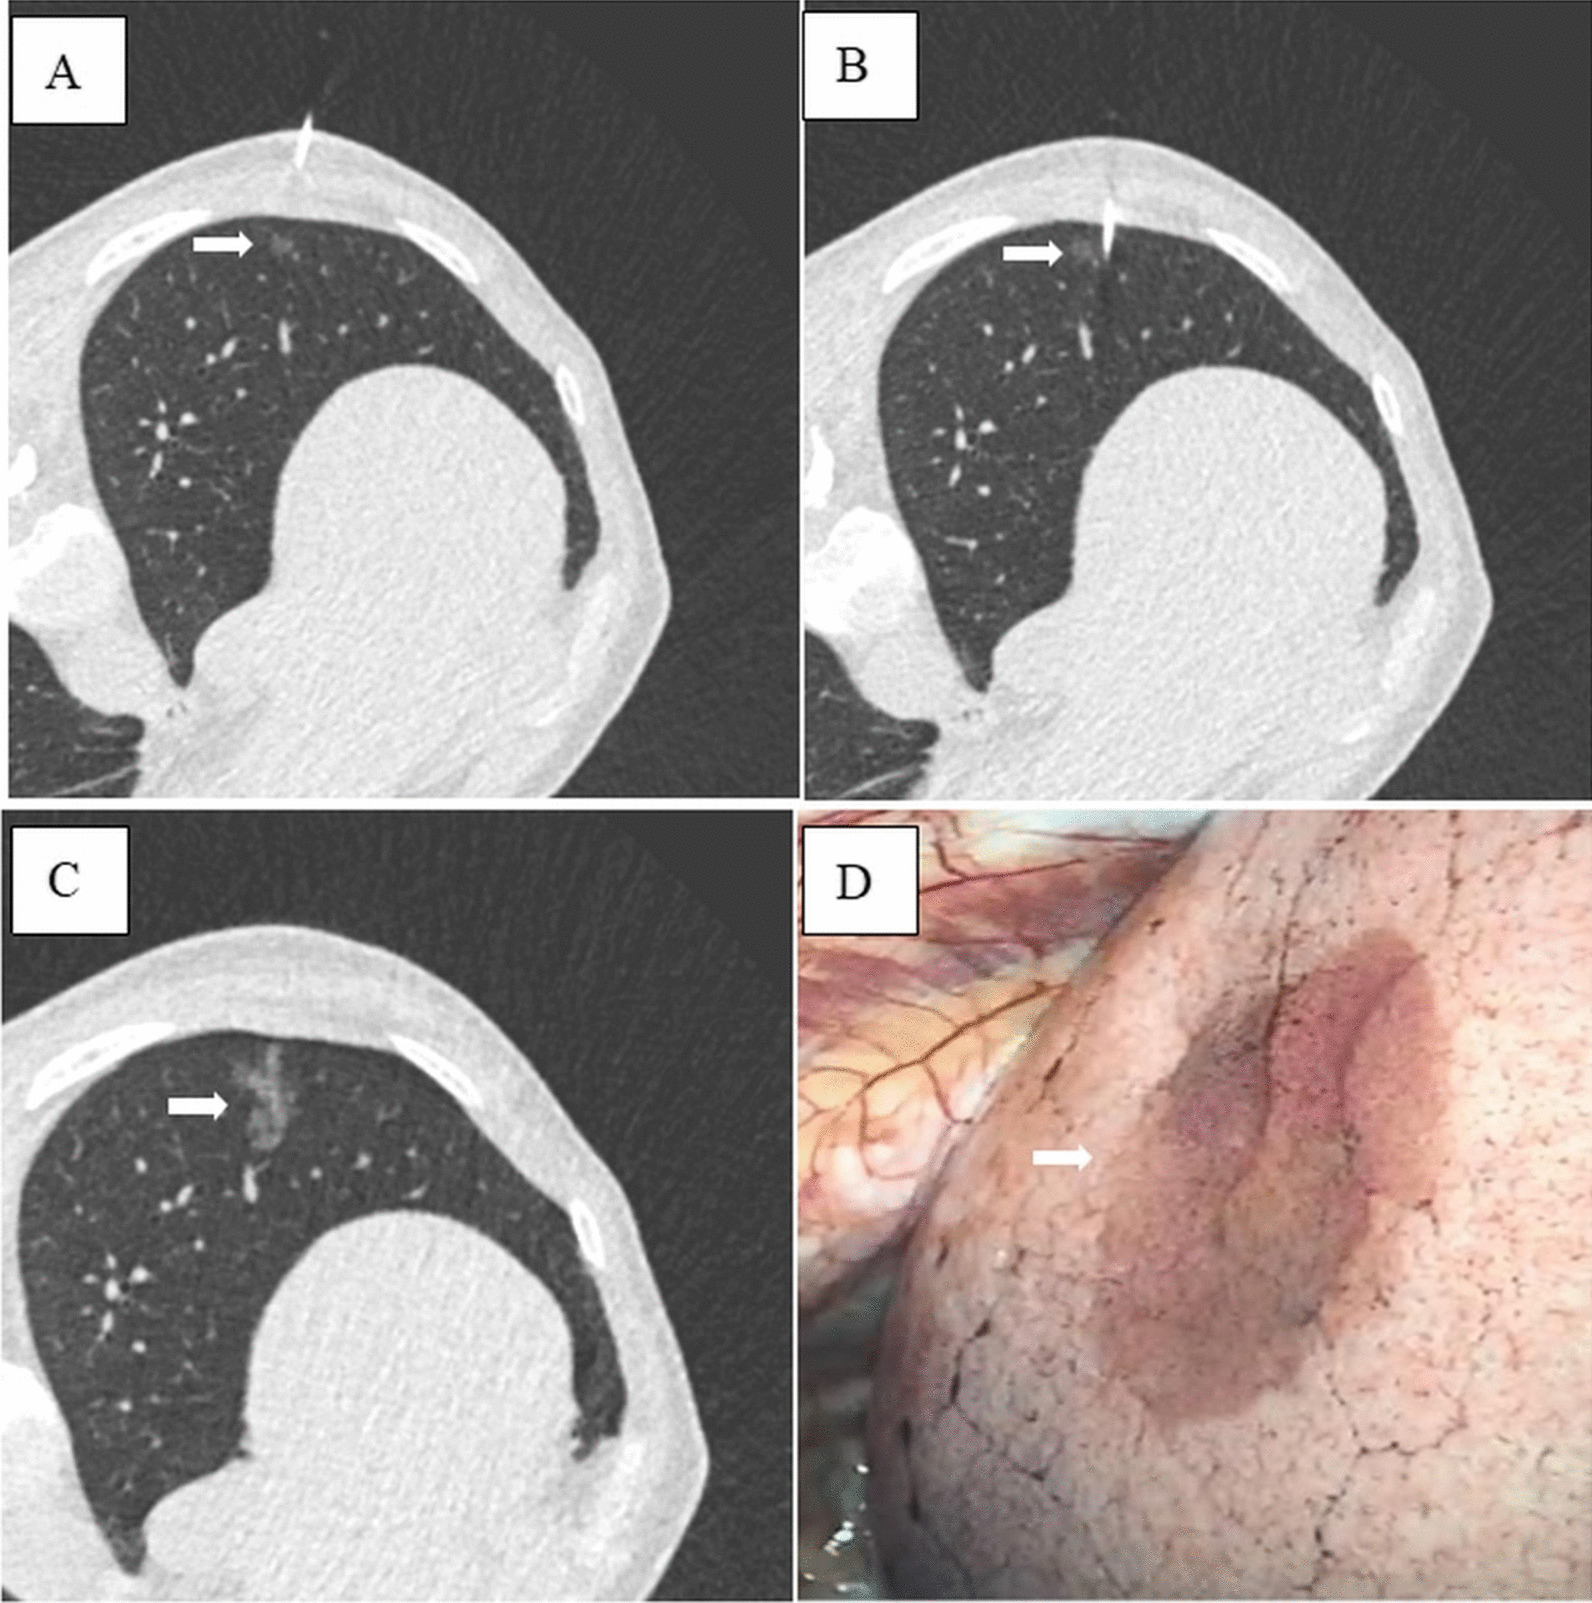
Computed tomography (CT)-guided autologous blood localization on a 65-year-old female with a 8 mm pure ground-glass nodule in the left lower lobe. A CT image showing a small pulmonary nodule (arrow) in the left lower lung lobe; B CT image showing the coaxial needle placement 5–10 mm caudal to the nodule (arrow); C CT image showing the change after the injection of the suspension of autologous blood (arrow); D hematoma on the pleura (white arrow) during video-assisted thoracoscopic surgery
